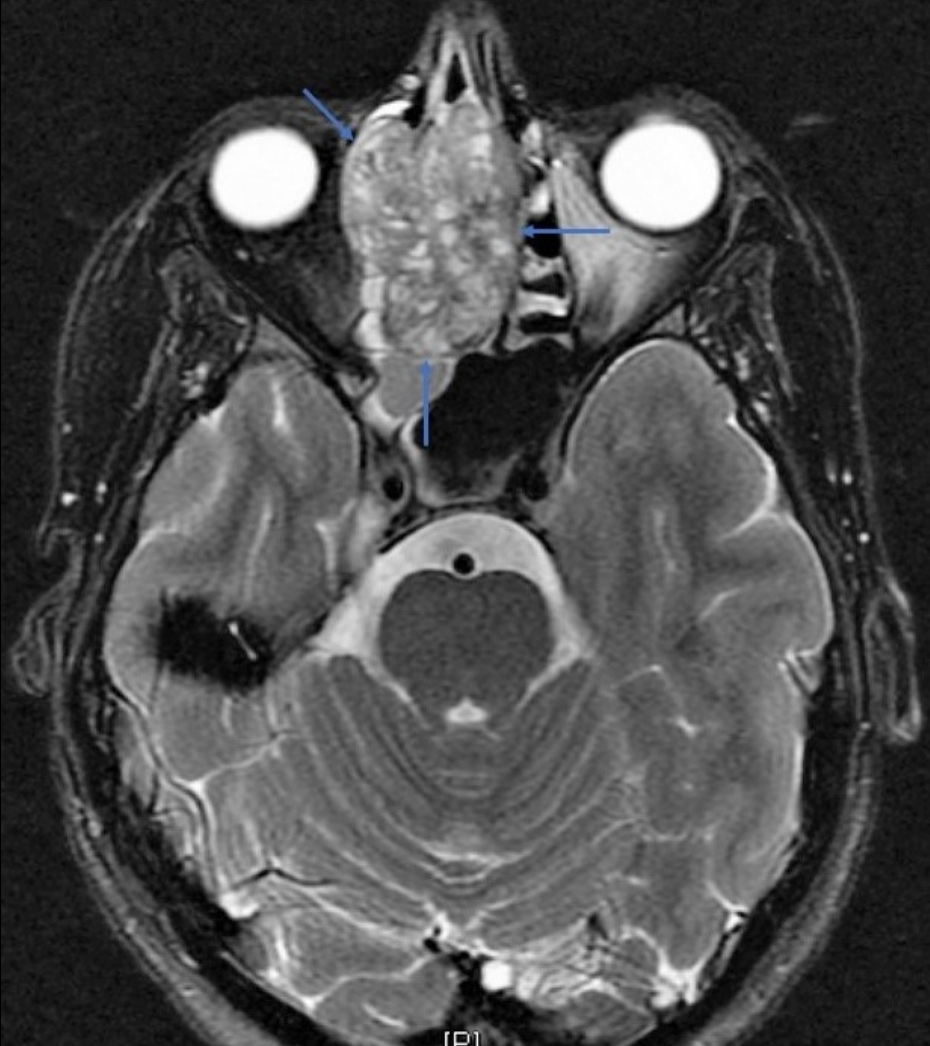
Transverse magnetic resonance imaging (MRI) without contrast confirms computed tomography findings and demonstrates extension of mass into extraconal right orbit
